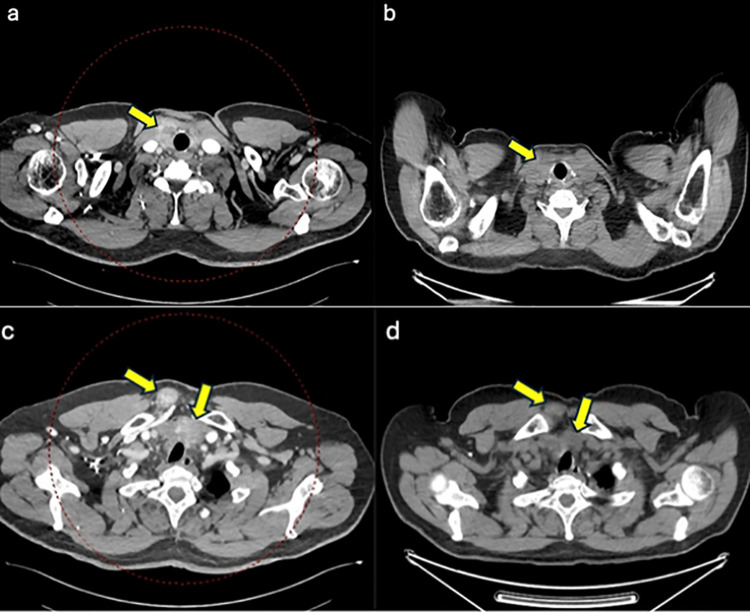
Representative pre- and post-treatment CT scans. (a) Contrast-enhanced CT (pre-treatment): The yellow arrow highlights heterogeneously enhancing pathological tissue in the right thyroid bed, in a patient with prior total thyroidectomy. The lesion (approximately 32 × 26 mm) infiltrates the prethyroid muscles and extends caudally into the anterior mediastinum, reaching a maximum size of 42 × 41 mm. (b) Post-treatment CT (non-contrast): The yellow arrow demonstrates a marked reduction in size and metabolic activity of the lesion, indicating a positive therapeutic response. No contrast-enhanced CT image was available at this time point because only PET/CT without intravenous contrast was performed for metabolic response assessment. (c) Contrast-enhanced CT (pre-treatment): The yellow arrows show additional pathological tissue in the presternal region (approximately 43 × 32 mm), infiltrating the right sternocleidomastoid muscle, which appears swollen compared to the opposite side. There is also posterior infiltration of the right half of the sternal manubrium, with focal cortical erosion. (d) Post-treatment CT (non-contrast): The yellow arrows indicate a significant reduction in both volume and metabolic activity of the pathological tissue, consistent with a good treatment response. Contrast-enhanced imaging was not available in the post-treatment setting, as PET/CT without intravenous contrast was used to monitor the metabolic outcome.
